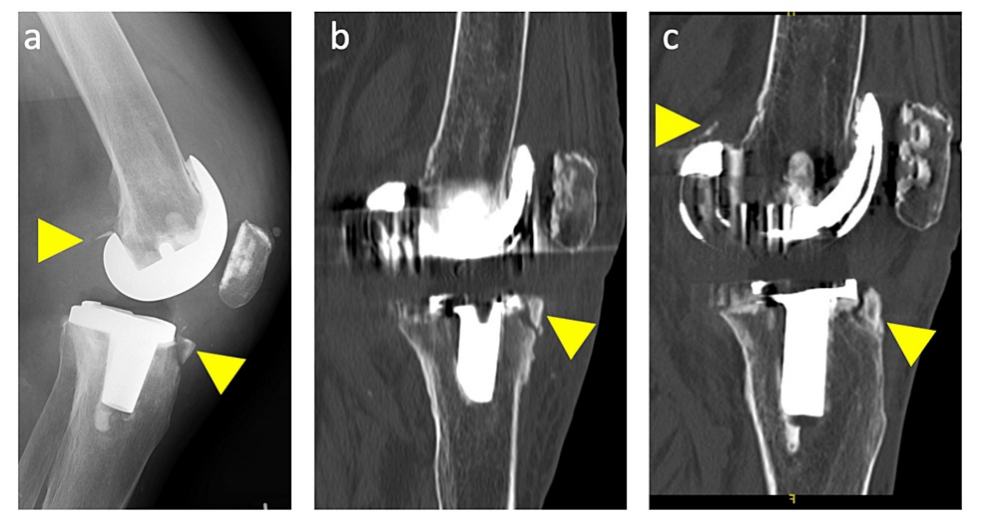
Knee images after fall. Lateral knee radiograph (a) and computed tomography images (b and c) after fall showed avulsion fractures (yellow arrows) at anterior aspect of the tibia and posterior aspect of the femur.
